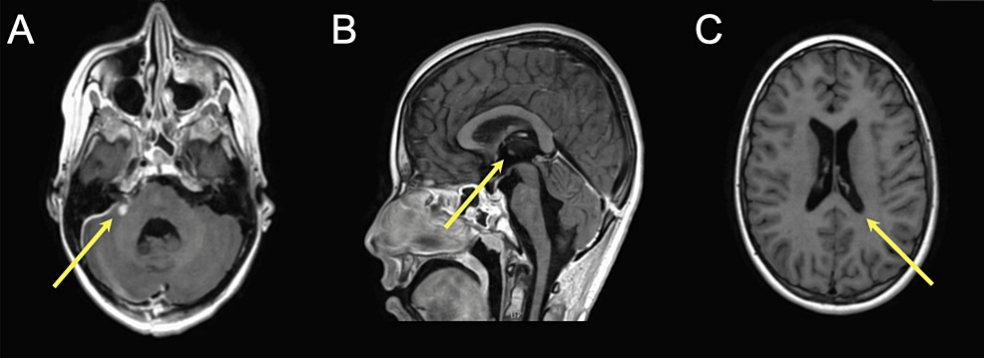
Repeat MRI on day 17 post-surgery showed regression of the cerebellopontine angle abscess (A) and hydrocephalus (B-C).
